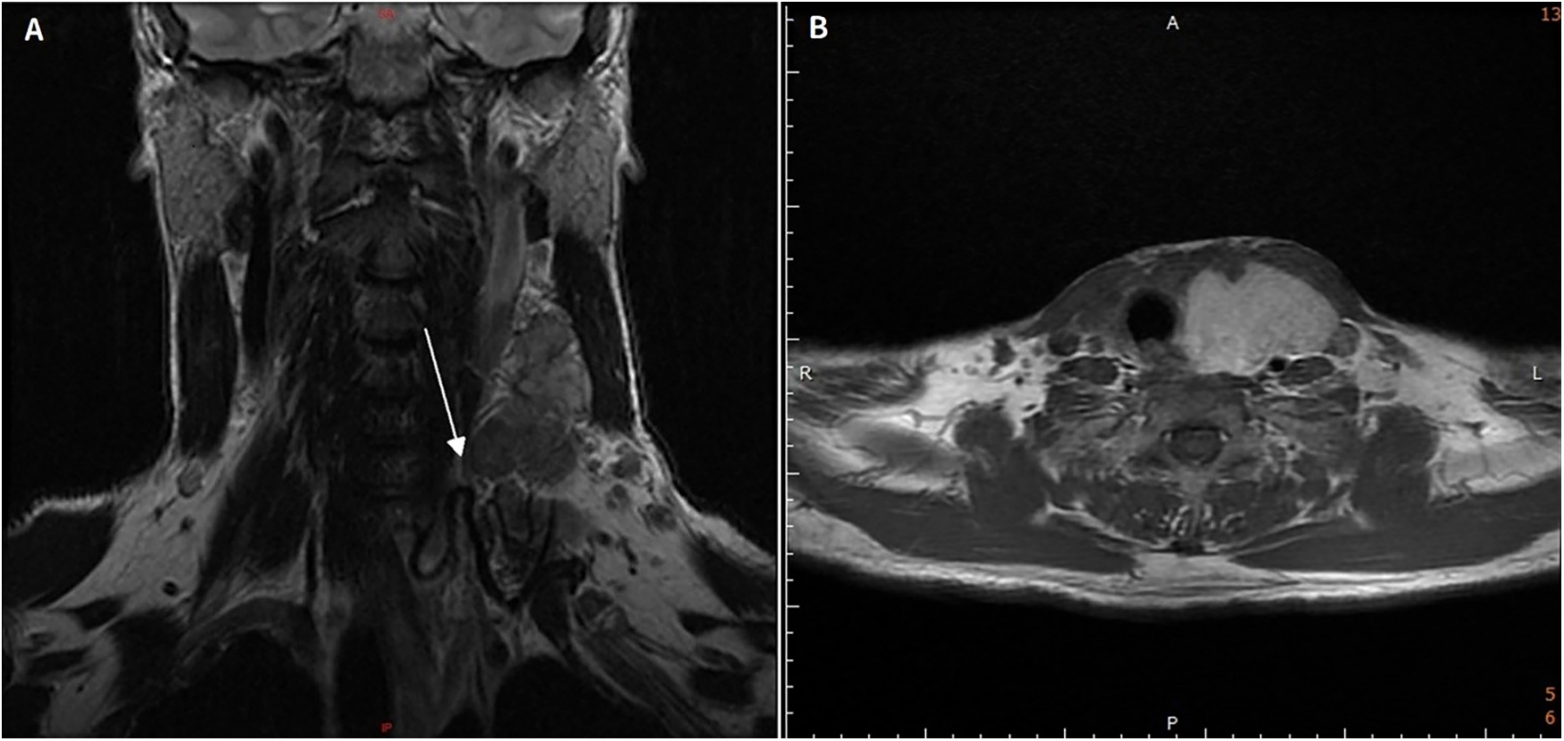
Pre-operative clinical and magnetic resonance images. (A,B) Showed a large cystic lesion with well-defined margins involving left thyroid gland, results in right deviation of the trachea.
